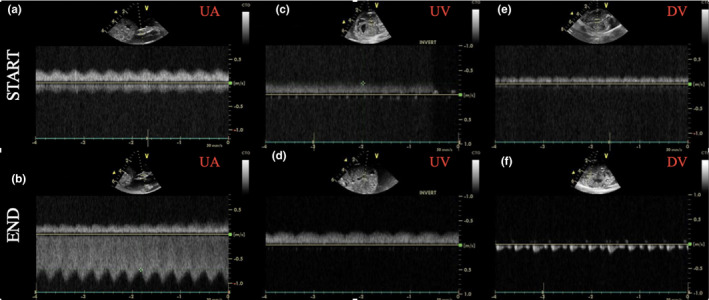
Pulsed Dopplers of the UA, UV and DV at the start and end of AP support. Example image of pulsed Dopplers of the UA (a,b), UV (c,d), and DV (e,f) at the start (a,c,e) and at the end (b,d,f) of AP support. At the start (within the first hour) of AP support UA, UV, and DV velocity waveforms were normal (a,c,e); however, toward the end (within the last 2 h) of the AP support waveform patterns became abnormal (b,d,f)
